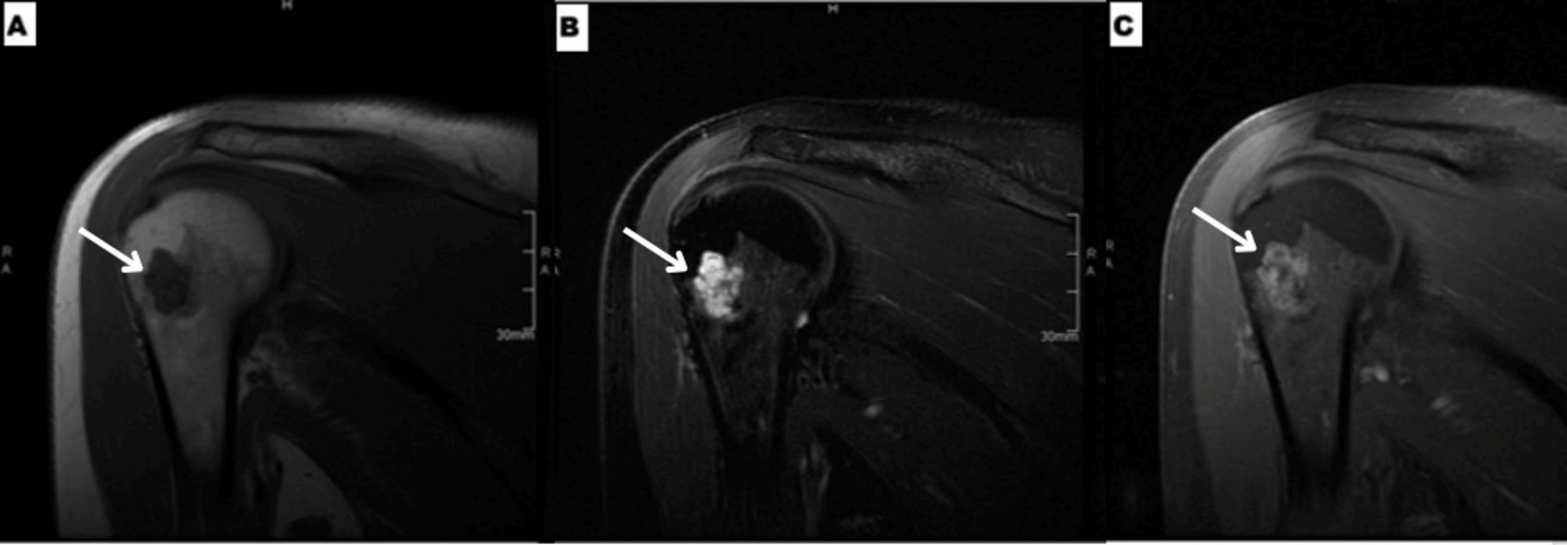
MRI findings - 1 2009 coronal T1 (A), coronal T2 fat sat (B), and coronal T1fat sat post contrast (C) MRI images show a well circumscribed, non-aggressive mildly lobulated lesion in the humeral head and neck central medullary space which is low in signal on T1, predominantly bright on T2 fat sat with internal low signal foci and shows mild predominant peripheral enhancement without endosteal erosion or perilesional marrow edema (arrows) MRI: magnetic resonance imaging
